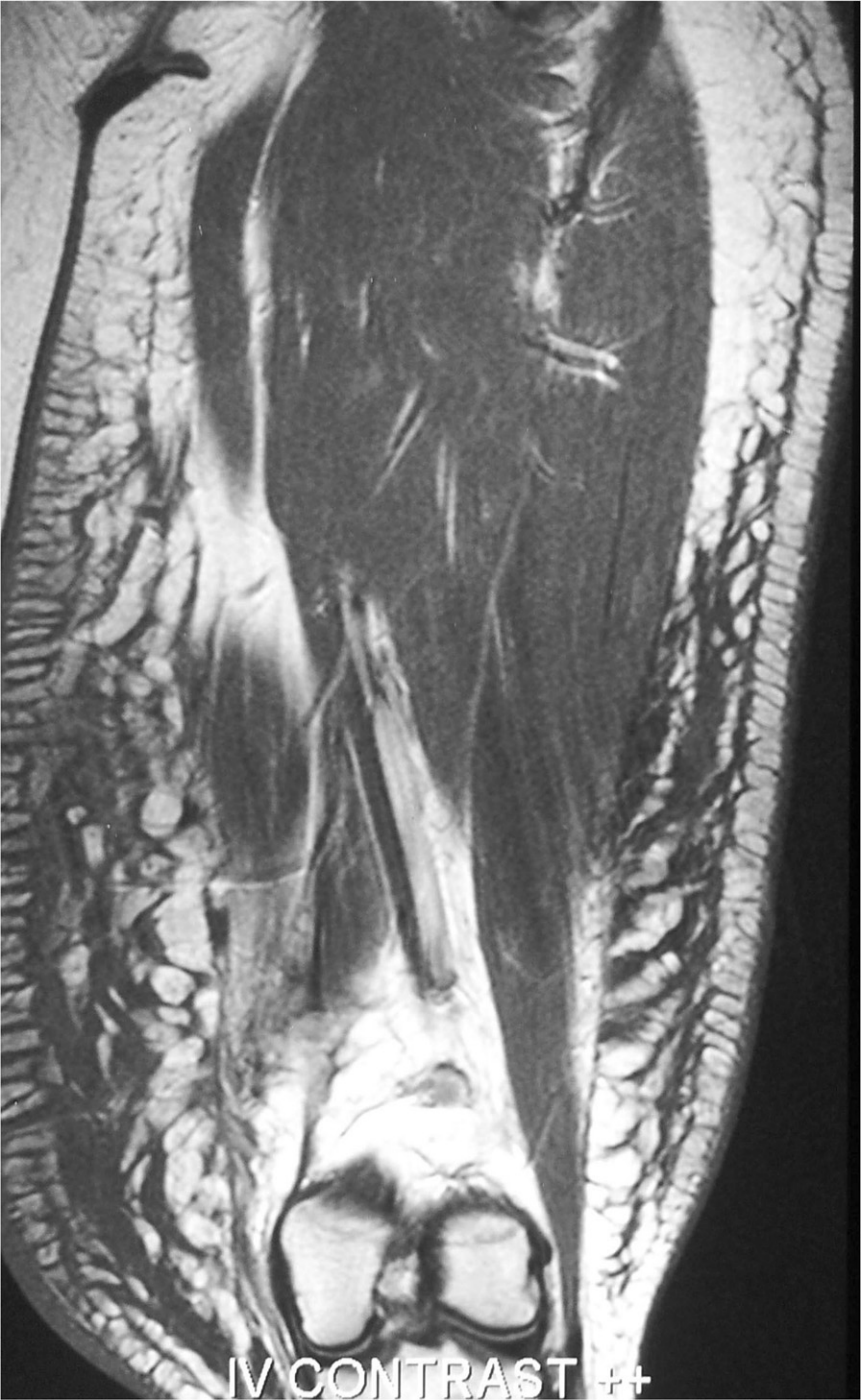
Magnetic resonance imaging of the thigh mass showing a sagittal section.
